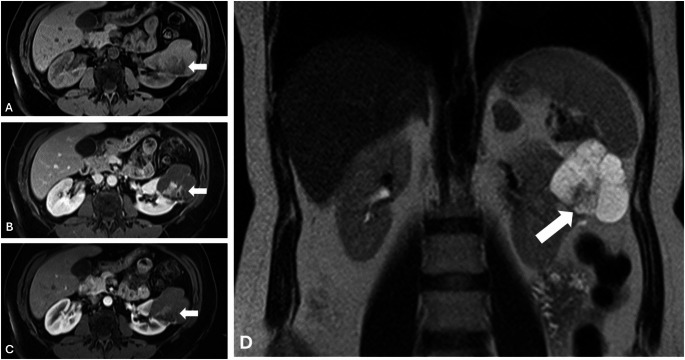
Complex renal cyst in a 54-year-old female with HLRCC. Contrast-enhanced MRI reveals a complex cyst measuring 5.8 cm, featuring a solid component (arrow) visible in the pre-contrast phase (A) and on coronal T2 images (D), with heterogeneous enhancement noted in the post-contrast nephrogenic (B) and delayed (C) phases. Final pathology report confirmed the cyst as malignant.
